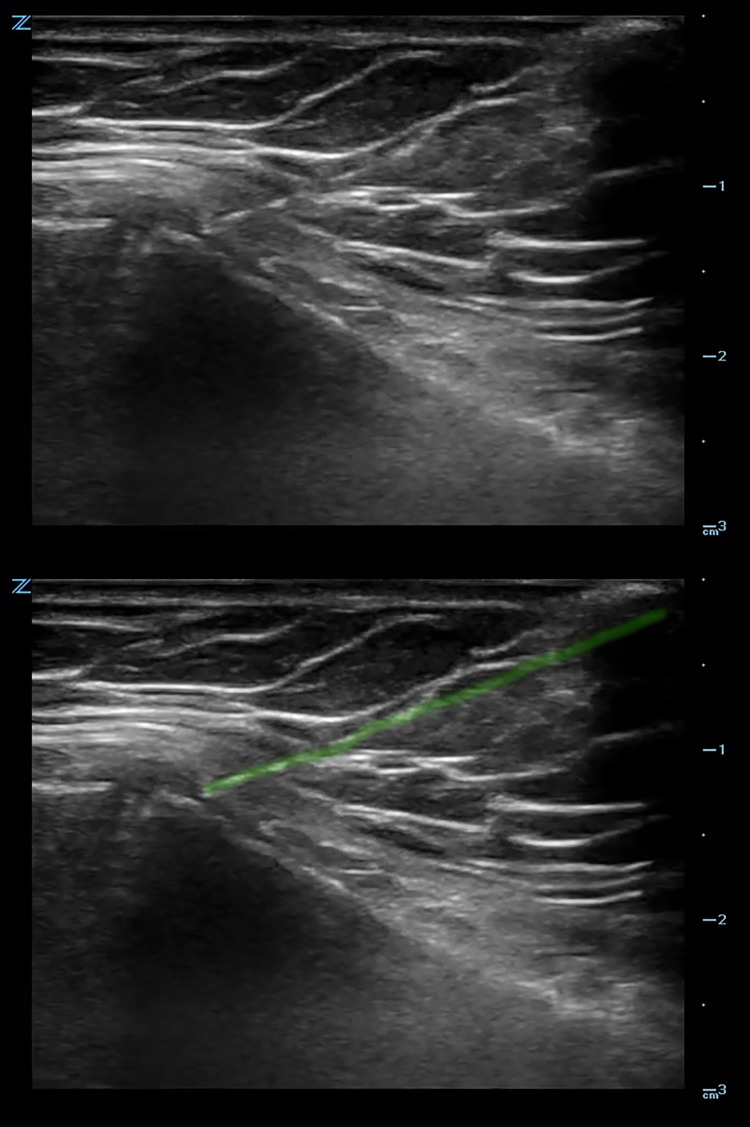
Ultrasound image of in-line needle insertion in the hematoma overlying the fracture. The needle is highlighted (green).
